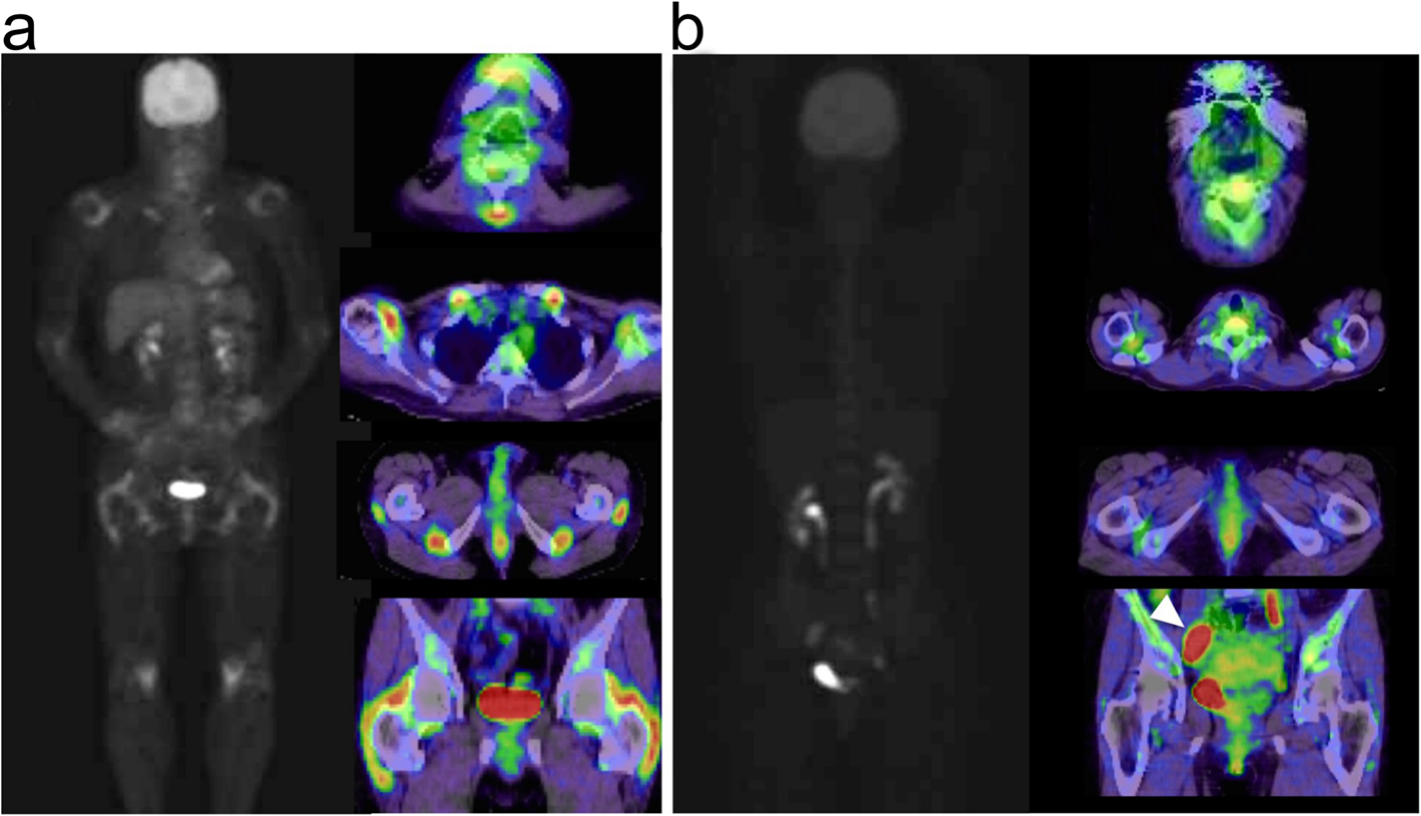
a Typical FDG–PET/CT findings in a patient with PMR. High FDG uptake seen in PMR-specific sites, including cervical and lumbar interspinous bursae, shoulders, sternoclavicular, and hip joints, greater trochanters, and ischial tuberosities. b FDG–PET/CT in a 50-year-old woman with ovarian cancer. Strong FDG uptake in the ovary (arrowhead). Weak FDG uptake in the shoulders but not in any other PMR-specific sites
